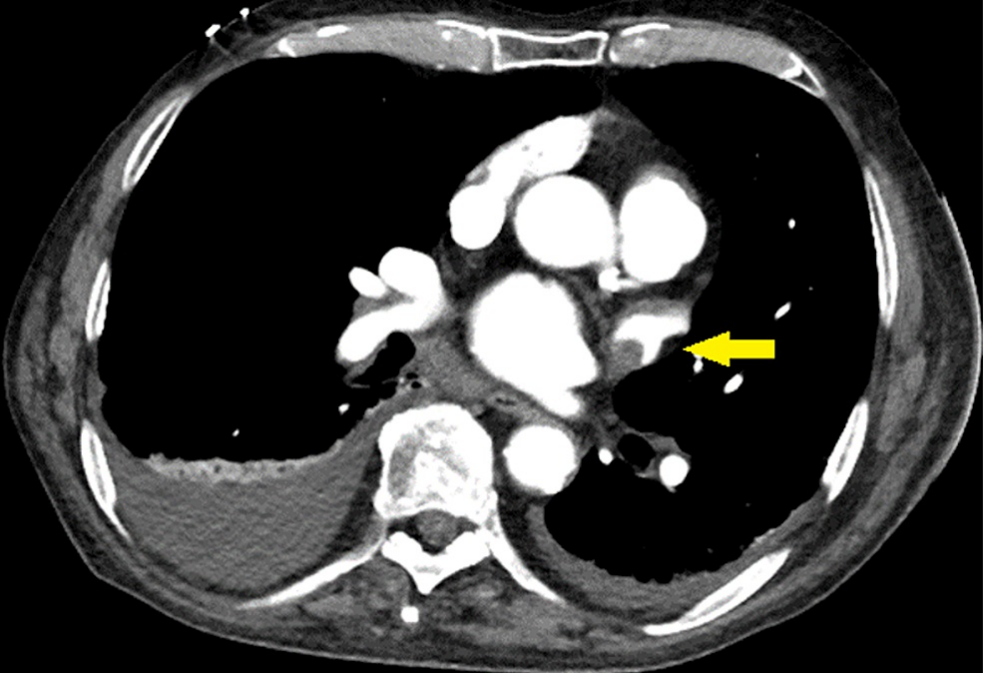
Computed tomography pulmonary angiography showing a left atrial appendage thrombus (yellow arrow).
